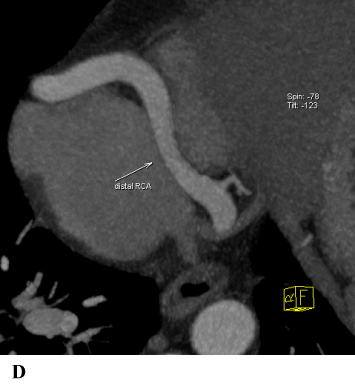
Cardiac multidetector computed tomography (MDCT) angiogram volume rendering reconstruction of cardiac structures (images A-B) and multiplanar reconstruction images (images C-D) showed a dilated, tortuous right coronary artery (RCA) that eventually gave off a fistulous connection into the right atrium (RA).*
